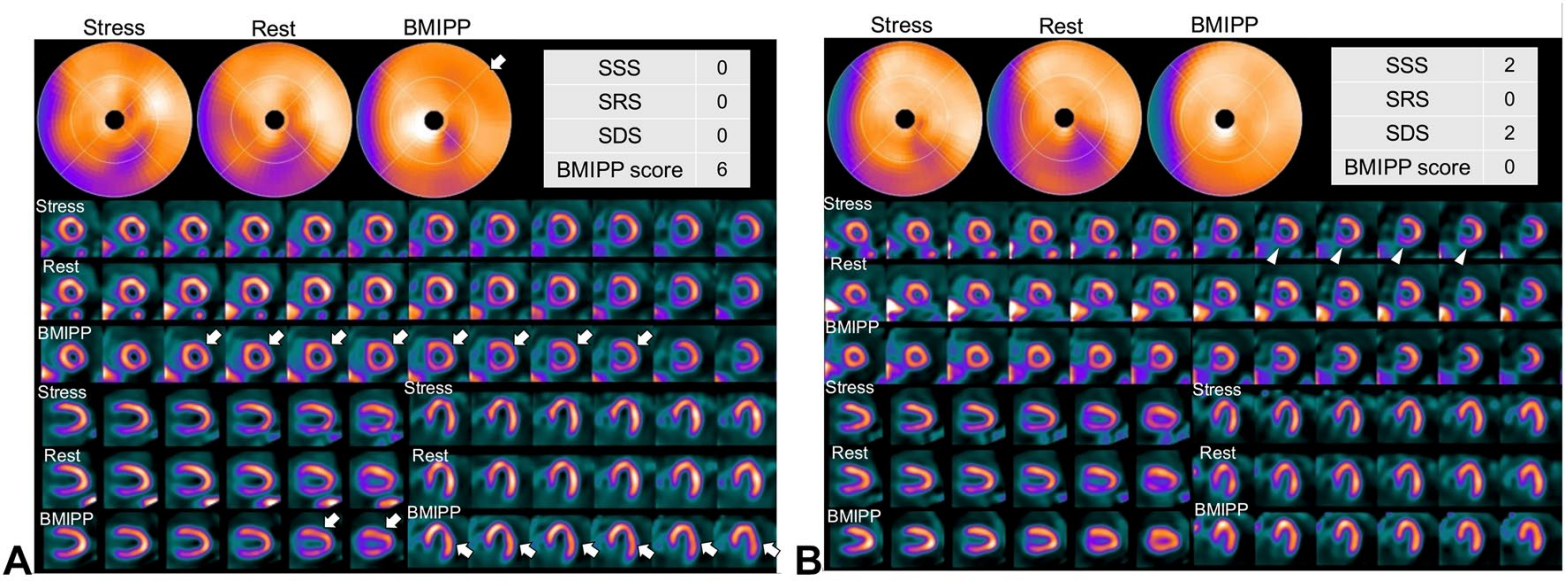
a At 3 months after CABG. ^99m^Tc-tetrofosmin pharmacological stress perfusion SPECT showed neither ischemia nor infarction. A decreased uptake in the inferior wall, which was more prominent at rest, was considered to be an artifact due to attenuation and/or high abdominal accumulation. In BMIPP SPECT, there was a mild to moderate decrease in anterolateral wall as scored BMIPP score 6 (white arrow). b At 25 months after CABG, anterolateral tracer activity of BMIPP SPECT was recovered. Inferior defect in perfusion SPECT at rest was considered to be an artifact due to high abdominal uptake. A slight posterior defect on stress images was also considered to be an artifact, however, was scored as SDS 2 (white arrowhead)
